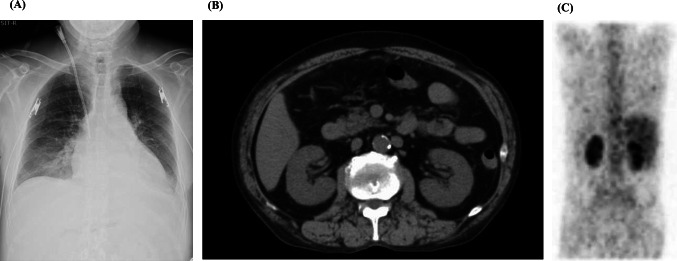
A Chest radiography shows decreased permeability of the lower lung fields in both lungs. B A CT scan shows that the kidney size is within the normal range. C Gallium scintigraphy shows abnormal accumulation of gallium in both kidneys, which is a finding of tubulointerstitial nephritis
